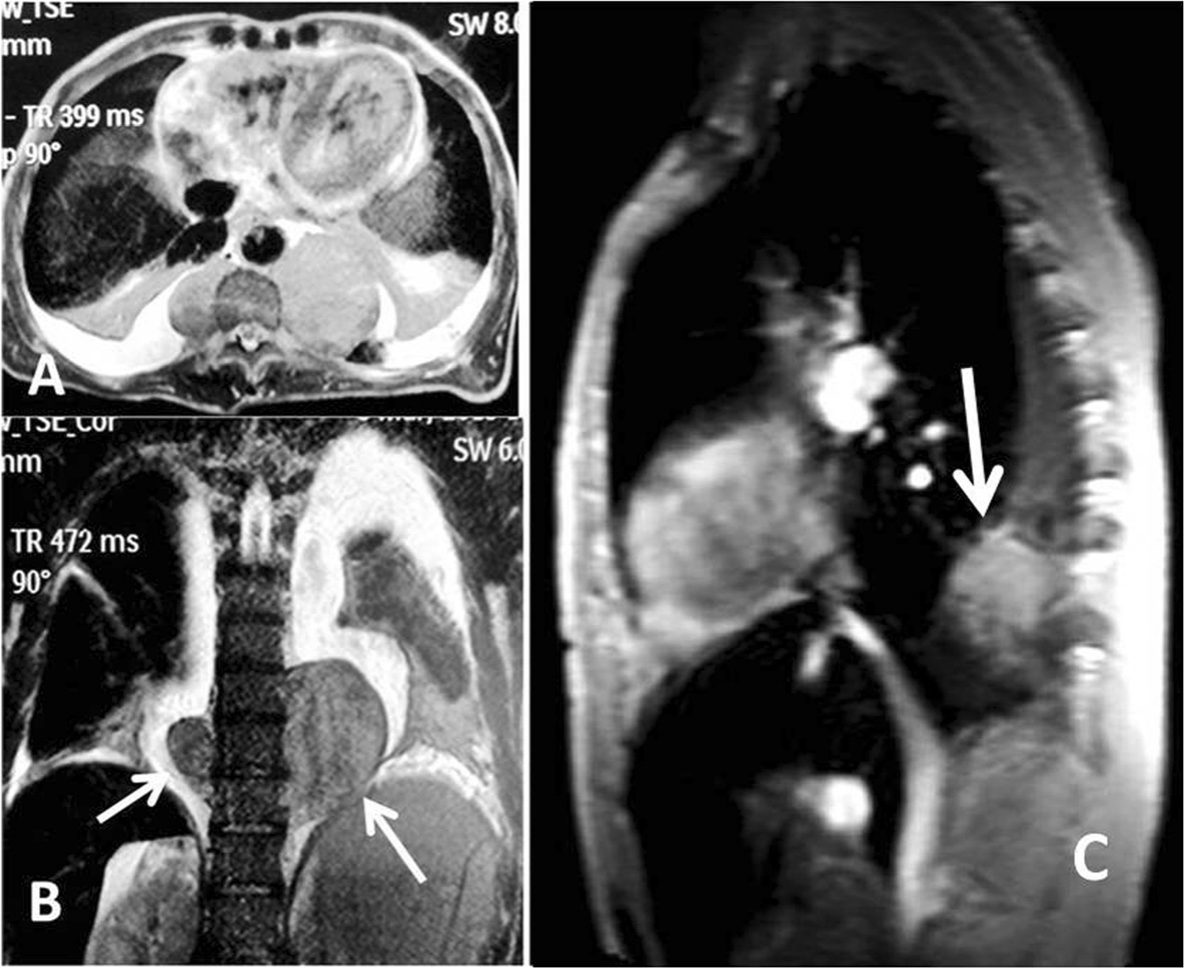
MRI features of the case. A: MRI scans reveals two masses in the posterior mediastinum. B: MPR on coronal plane shows a bilateral thoracic paravertebral mass. C: MPR on sagittal plane confirms the left mass in the posterior mediastinum.
